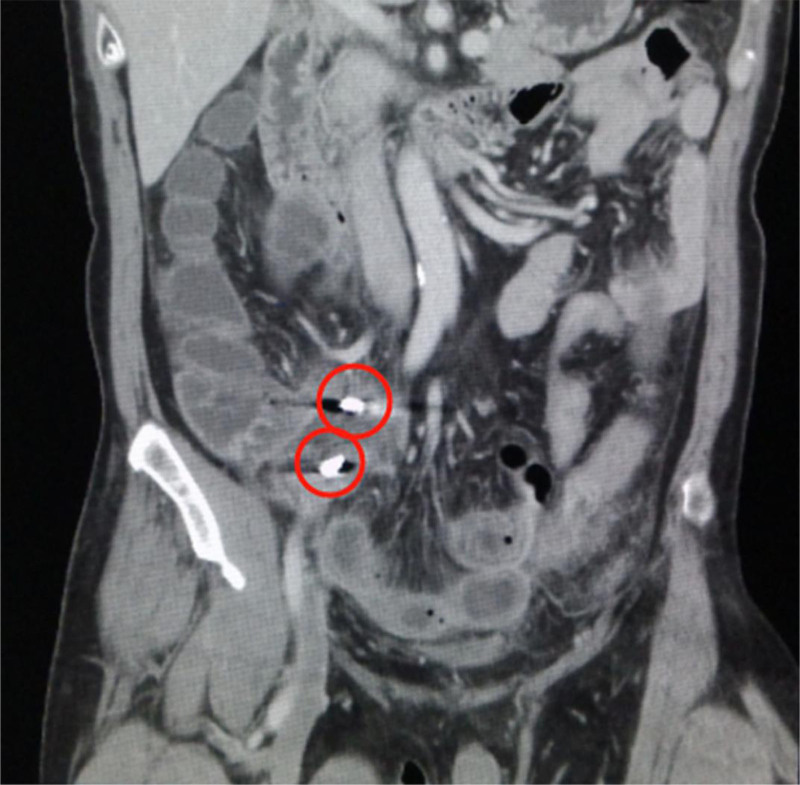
Computed tomography scan showing metallic objects around the appendix.
